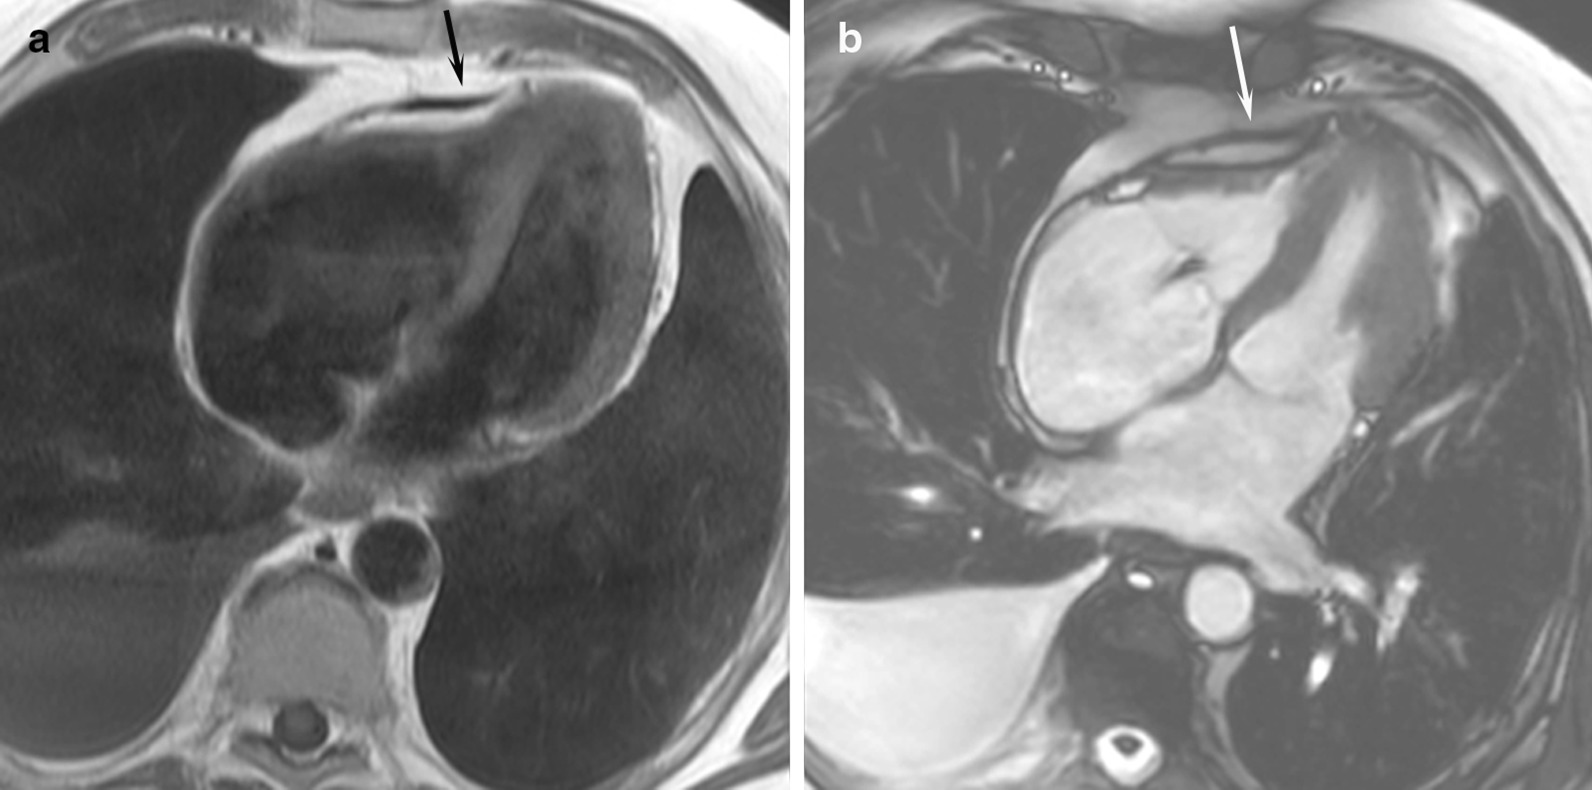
This 72-year-old male was admitted with dyspnea, pleural fluid and cirrhosis. Horizontal long-axis T1-weighted fast spin echo imaging shows (a) shows a focally thickened pericardium (black arrow) focally constricting the apical half of the right ventricle (RV). Horizontal long-axis cine imaging (b) confirms the constriction of the RV apex by the thick pericardium (white arrow). Presence of a moderate tricuspid regurgitation, bilaterally dilated atria, and right-sided pleural effusion, indirect findings compatible with constrictive pericarditis. The patient underwent successfully a pericardectomy
